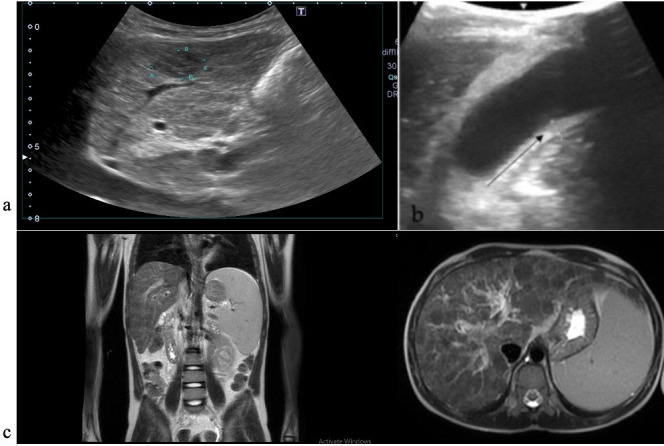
Imagistic findings in case 1. (a) Abdominal US—cirrhotic liver nodules. (b) Abdominal US—thick-walled gallbladder. (c) Abdominal MRI—enlarged liver and spleen.
